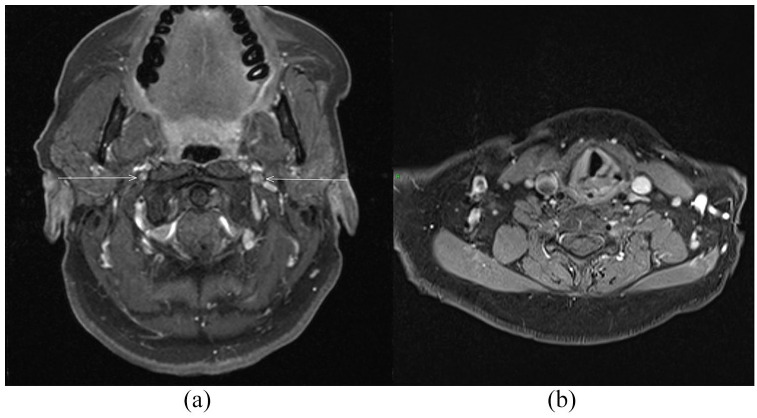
(a) Bilateral thickening enhancement of the glossopharyngeal and vagus nerves (arrows) on MRI scan (axial T1-weighted fat saturated post contrast sequence). (b) Left vocal cord palsy seen on MRI scan (axial T1-weighted fat saturated post contrast sequence).
